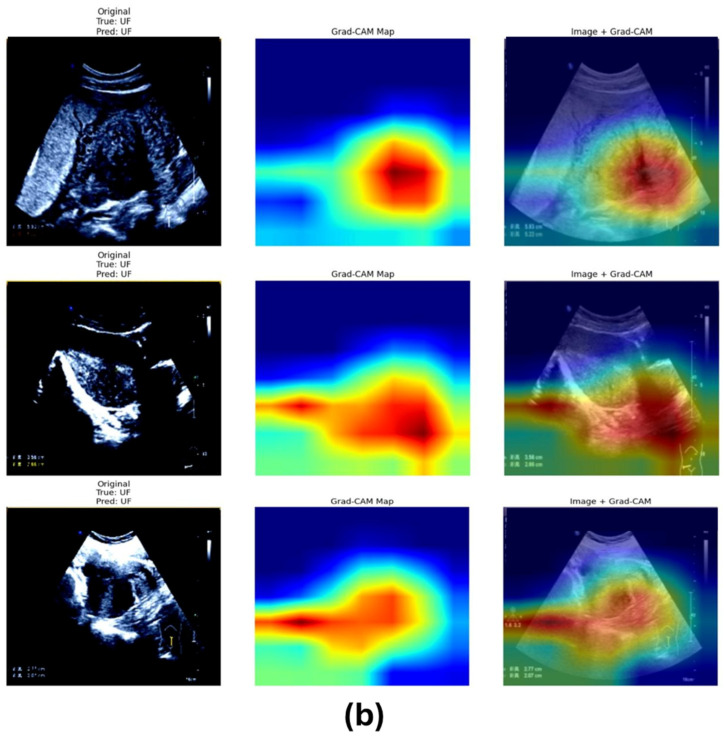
LIME-based heatmaps of ultrasound image test samples: (a) normal uterine conditions (NUF) show mild and scattered activation, while (b) fibroid conditions (UF) show strong and concentrated activation over the fibroid tissue itself. This confirms that the model focuses precisely on the disease and is used for diagnosis.
